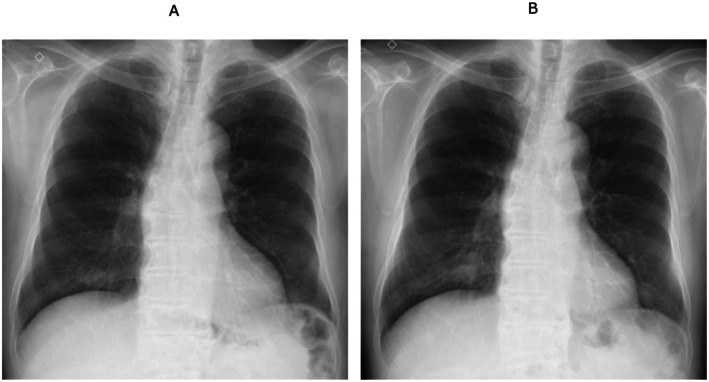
Chest radiographs. (A) Chest radiograph obtained at the initial presentation showing multiple nodular opacities in both lungs, predominantly in the lower lung fields. (B) Follow‐up chest radiograph obtained after the initiation of antimicrobial therapy showing interval changes in the pulmonary nodules, corresponding to the heterogeneous evolution observed on CT.
